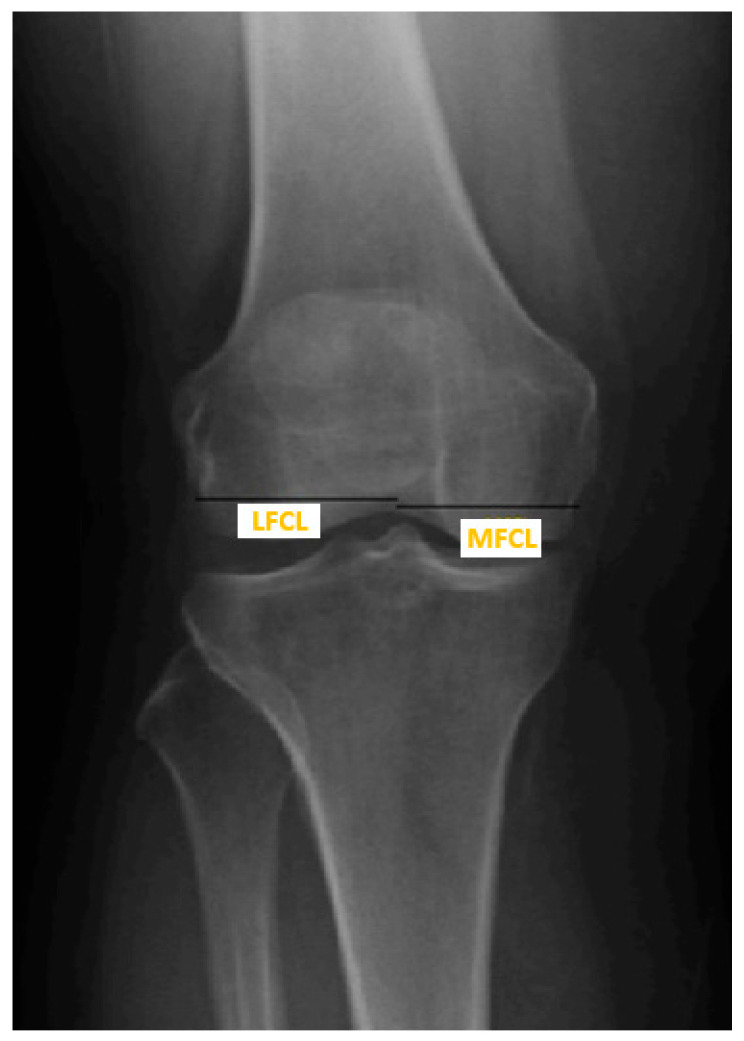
The lateral and medial femoral condyle lengths were measured on the plain radiograph.
